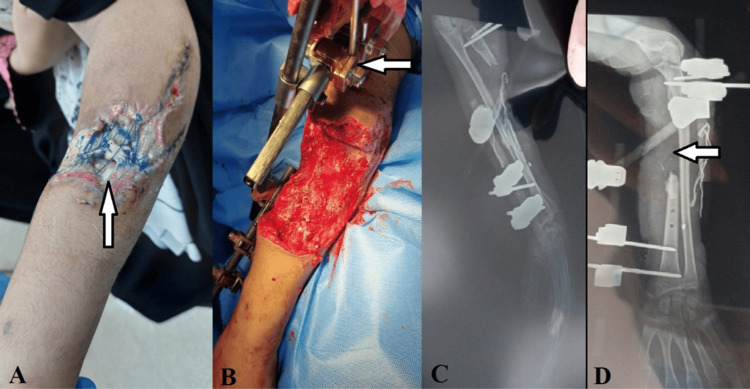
Skin necrosis, repeat debridement, and external fixation (A) Two-week follow-up clinical photograph demonstrating the extent of skin necrosis and the soft-tissue defect. (B) Intraoperative photograph following the second debridement, with removal of the plate and application of an external fixator, showing the extent of soft-tissue loss. (C, D) Postoperative anteroposterior (D) and lateral (C) radiographs demonstrating external fixation and the bone defect following plate removal.
